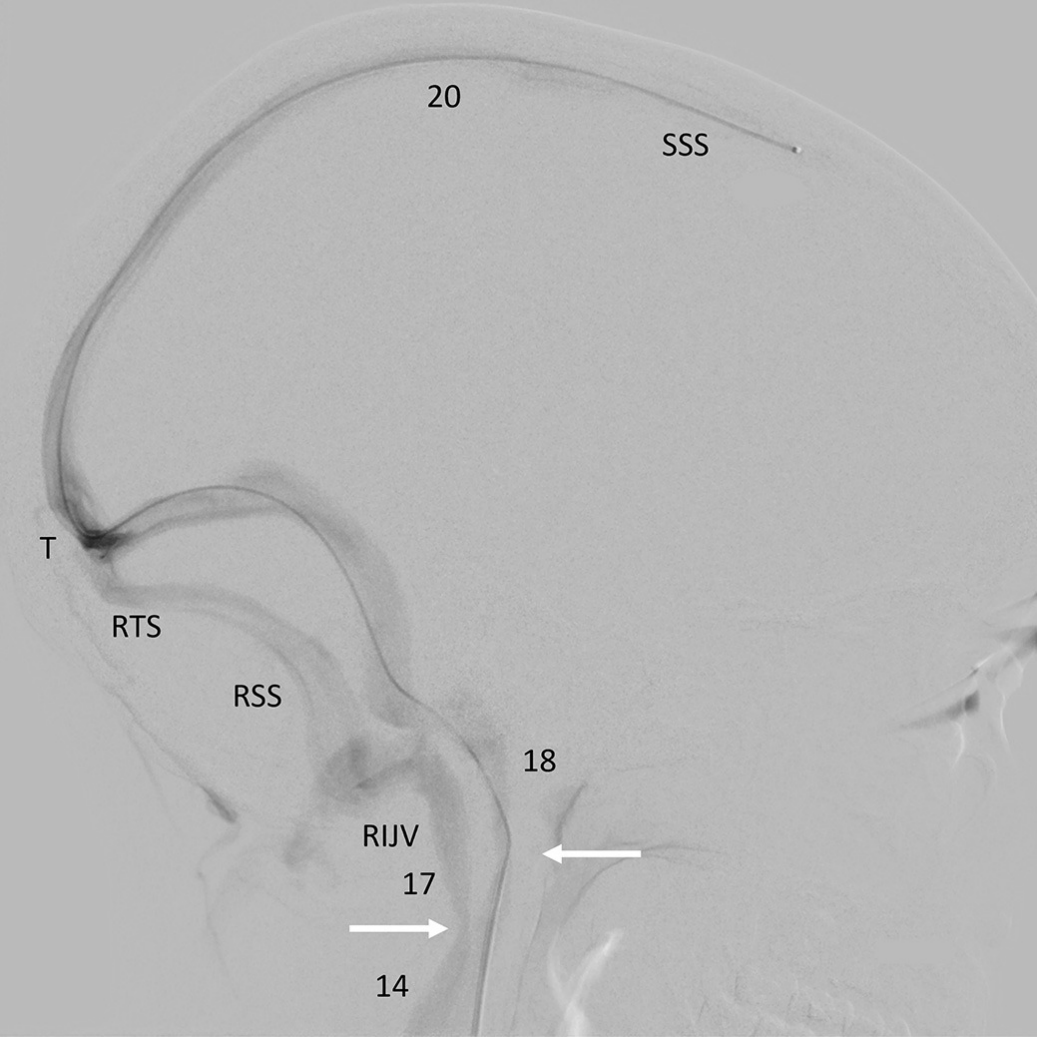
Catheter venogram. Background-subtracted, oblique lateral view of the head and upper neck. Radiographic contrast injected through a catheter passed up through the left lateral sinus into the sagittal sinus outlines the sagittal, both lateral sinuses and upper jugular veins. There is a narrowing of both internal jugular veins (arrows) where they pass over the transverse processes of C1 (SSS, superior sagittal sinus; T, torcula; RTS, right transverse sinus; RSS, right sigmoid sinus; RIJV, right internal jugular vein) (Numbers = pressure recordings in cm H_2_O).
